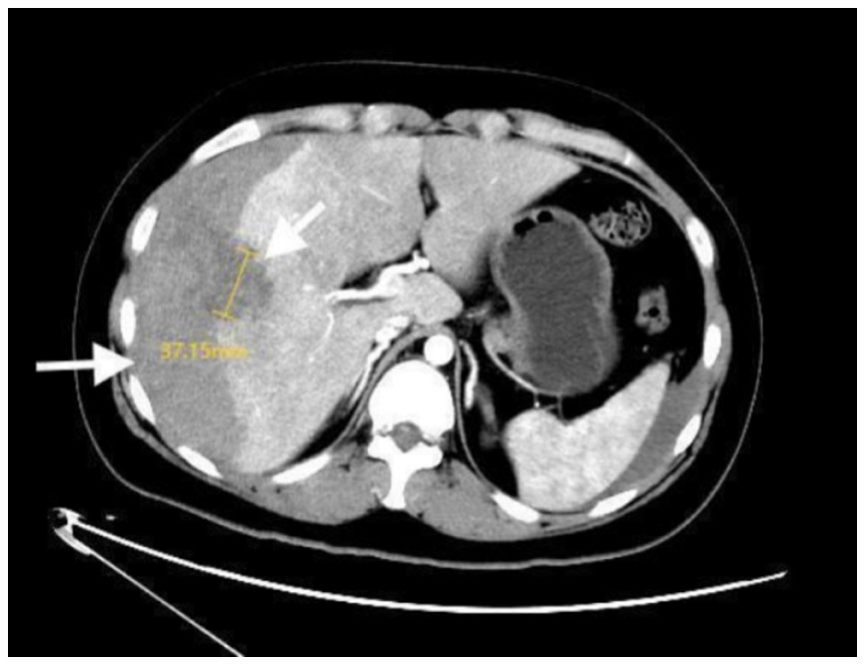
Contrast-enhanced abdominal CT images obtained on admission. Contrast-enhanced abdominal CT shows a 37.15 mm right-lobe abscess (left arrow) with massive subcapsular hematoma (right arrow).
